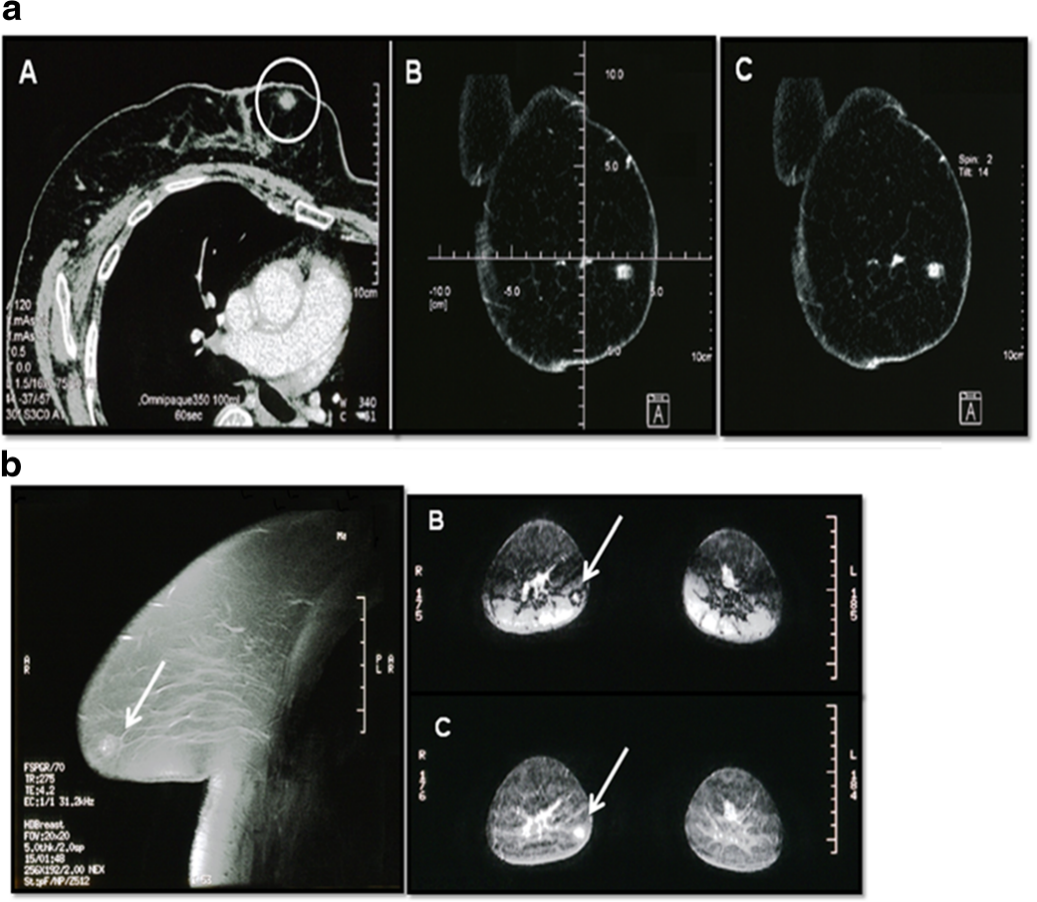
Enhanced computed tomography and magnetic resonance imaging findings. a. Enhanced computed tomography showed a well-enhanced nodule in both the early and late phase. b. Dynamic T-1 weighted image revealed a highly intense nodule in the right breast (A). A subtraction image showed a hyper-intense nodule in early and late phases (B, C).
